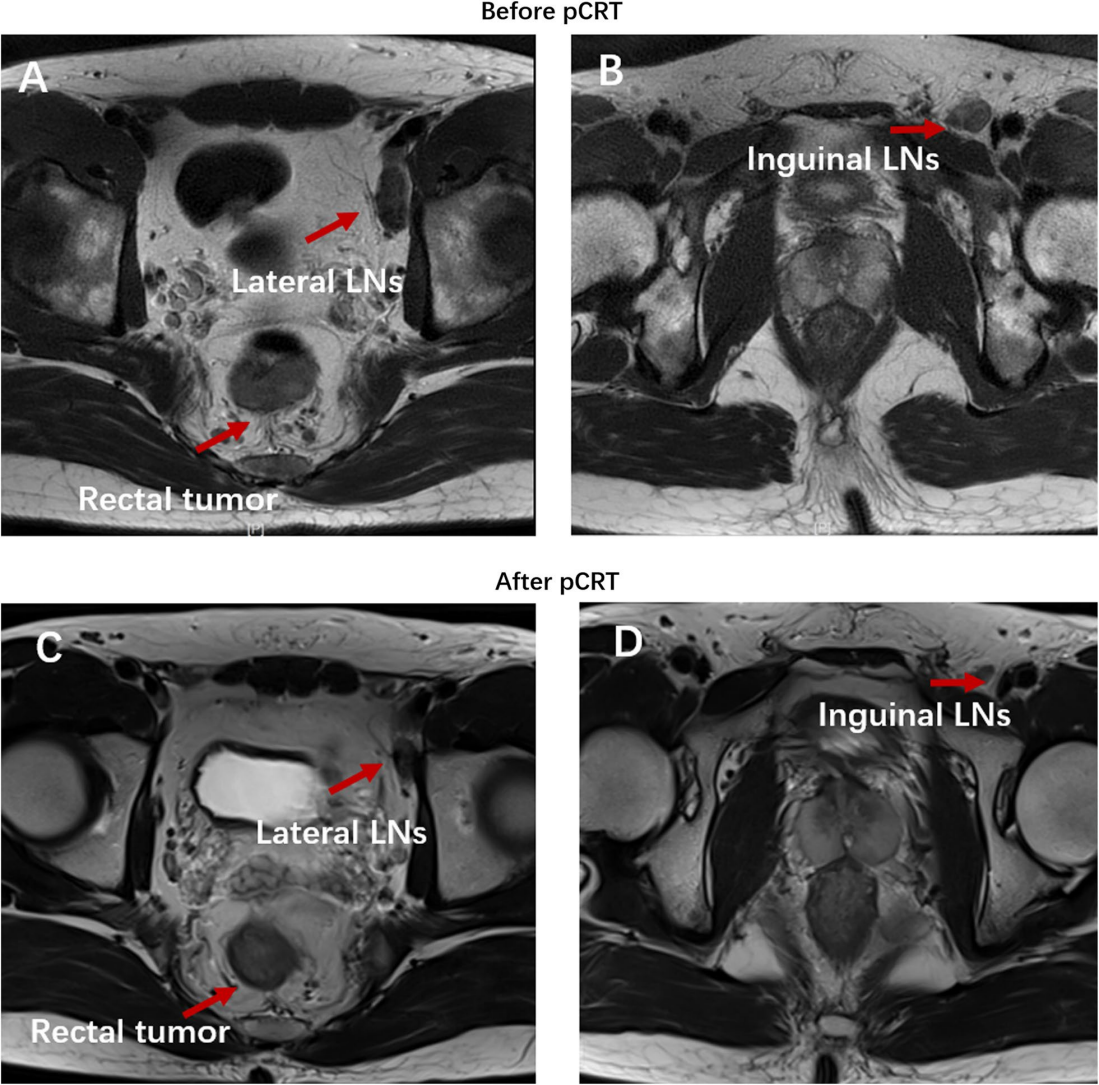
Pelvic MRI findings before and after pCRT in the high-resolution T2w sequences using a 3.0 Tesla Siemens Prisma MRI Scanner (Siemens Healthineers AG, Erlangen, Germany) with a contrast of Gadobutrol Injection (Gadovist). A Pelvic MRI before pCRT showing rectal tumor and left enlarged lateral LNs (axial T2WI sequence, no fat suppression, oblique transverse section, TR 2000, TE 95, FOV 20×20); B Pelvic MRI before pCRT showing left enlarged inguinal LNs (axial T2WI sequence, no fat suppression, oblique transverse section,TR 2000, TE 95, FOV 20×20); C After pCRT the rectal tumor and left enlarged lateral LNs decreased in size (axial T2WI sequence, no fat suppression, oblique transverse section, TR 2000, TE 89, FOV 22×22); D After pCRT the left enlarged inguinal LNs decreased in size (axial T2WI sequence, no fat suppression, oblique transverse section, TR 2000, TE 89, FOV 22×22). *MRI*: magnetic resonance imaging; *LNs*: lymph nodes; *pCRT*: preoperative chemoradiotherapy; *T2WI*: T2 weighted imaging; *TR*: repetition time; *ET*: echo time; *FOV*: field of view
